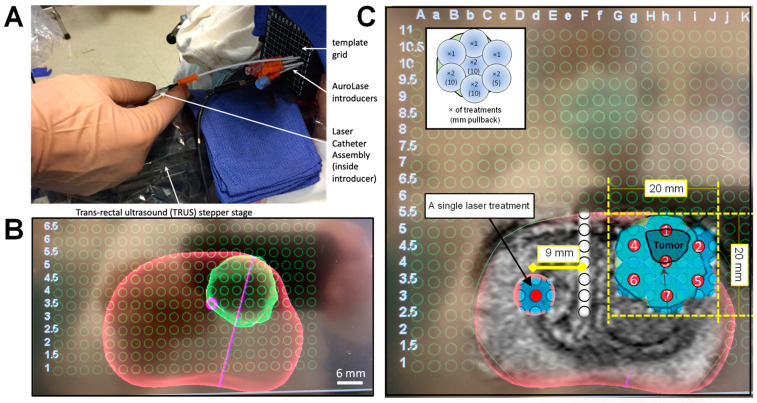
Laser treatment sequence under live MR/ultrasound fusion (MR/US) guidance. (A) The AuroLase^®^ procedure showing a Laser Catheter Assembly inside the cannula of the trocar introducer, in which the lateral position is fixed by the transperineal template grid. (B) A cropped screenshot from the UroNav screen showing the 3-dimensional (3D) prostate surface rendered image post-MR/US after the TRUS and template grid had been registered in 3D. The screenshot highlights the focal ablation volume (green: lesion plus ~7 mm margin) within the prostate gland (red). (C) An axial *T*_2_w MR image overlaid on the corresponding ultrasound image, visualizing how the two imaging modalities assist in localizing the tumor position in 3D space relative to the prostate gland. The prostate (red), ablation zone (tumor + margin: dark and light blue), and laser catheter insertion positions (numbered circles) are superimposed on the grid and represent the laser catheter insertion sequence. At the end of the treatment, an additional treatment [control burn] was performed in the contralateral hemisphere ~0.9 cm far from the urethra (U). The inset shows the number of laser treatments at each position in the treatment zone and the pullback distance (in mm) as needed to cover the longitudinal space of the targeted ablation volume. Based on this plan, the expected ablation dimensions were 2.0 cm × 2.0 cm (in-plane), 2.8 cm (extent).
